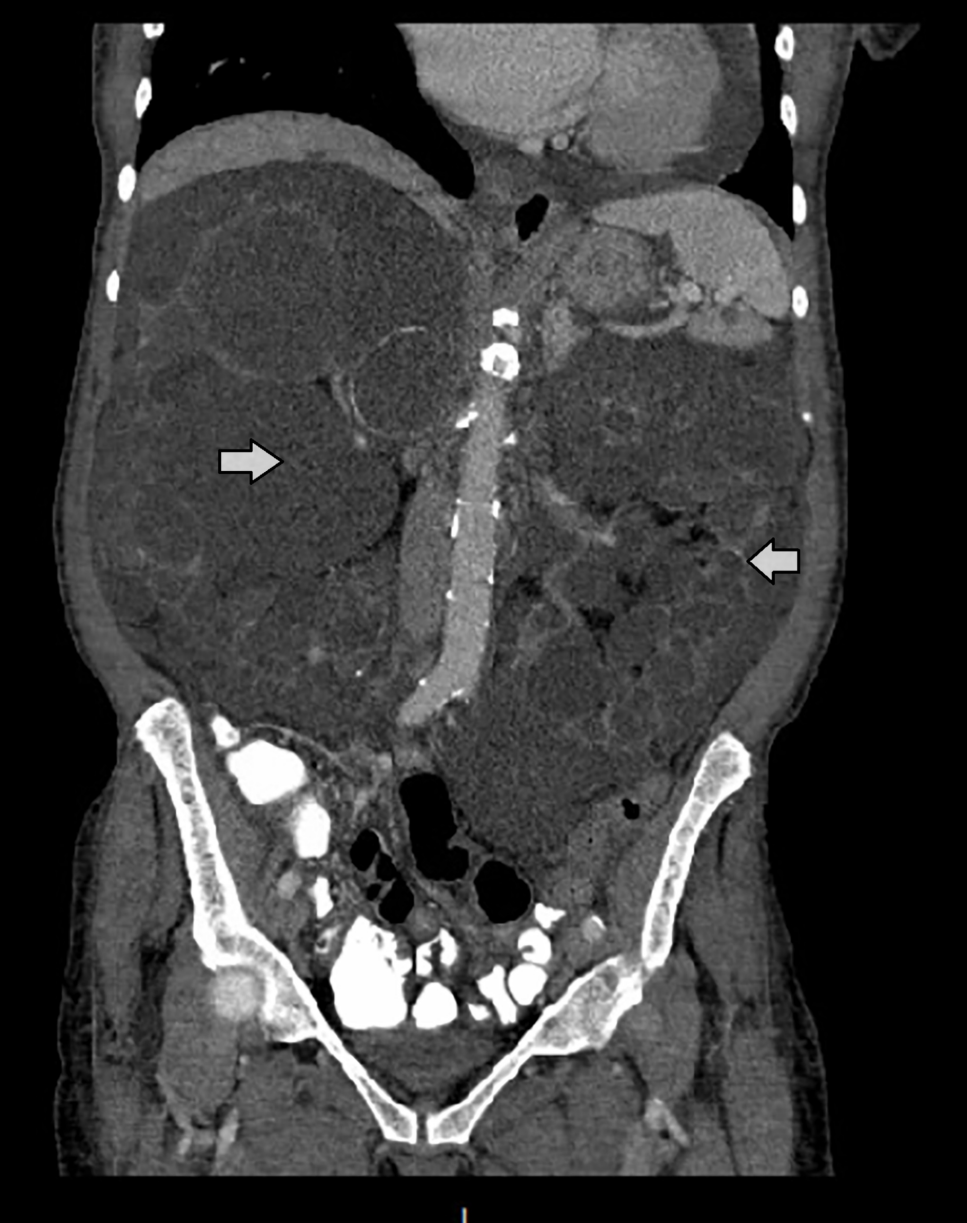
CT abdomen showing the severe cystic appearance of both kidneys (white arrows) CT: computed tomography
